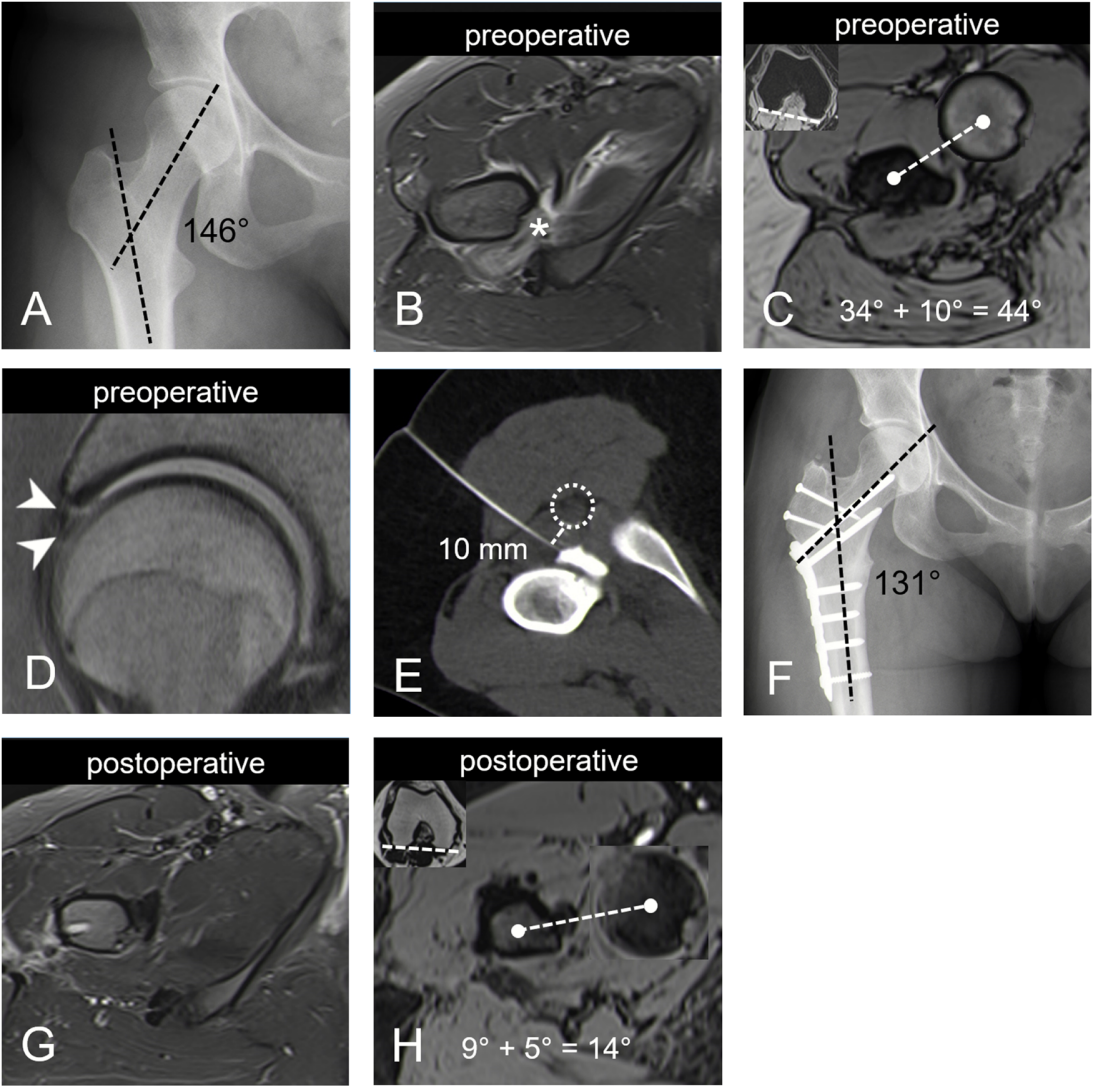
Anteroposterior pelvic radiograph of a 24-year-old female patient with right buttock pain and positive posterior impingement test on clinical examination. Diagnostic imaging revealed (A) a valgus deformity of the right hip on radiographs, (B) narrowing of the IFS with concomitant QFM edema (asterisk) on a preoperative axial short-tau inversion recovery MR-image, (C) increased femoral torsion (44°) measured on axial T1-w volume-interpolated breath-hold examination DIXON MR-image, as well as a (D) concomitant intrasubstance tear of the anterosuperior labrum (arrowheads) on a sagittal proton-density weighted turbo spin echo MR-image. This led to the clinical suspicion of IFI. The patient underwent (E) CT-guided diagnostic infiltration of the IFS in the prone position from a posterolateral approach with immediate post-procedural pain relief. The distance between the needle trajectory and the ischial nerve (dotted circle) was 10 mm (dashed line). Subsequently, (F) the patient underwent surgical hip dislocation with additional intertrochanteric varisation-derotation osteotomy with relief of symptoms. Postoperative MR-imaging following hardware removal performed for evaluation of the contralateral hip revealed a (G) normalization of the IFS and (G) corrected femoral torsion (14°, H)
